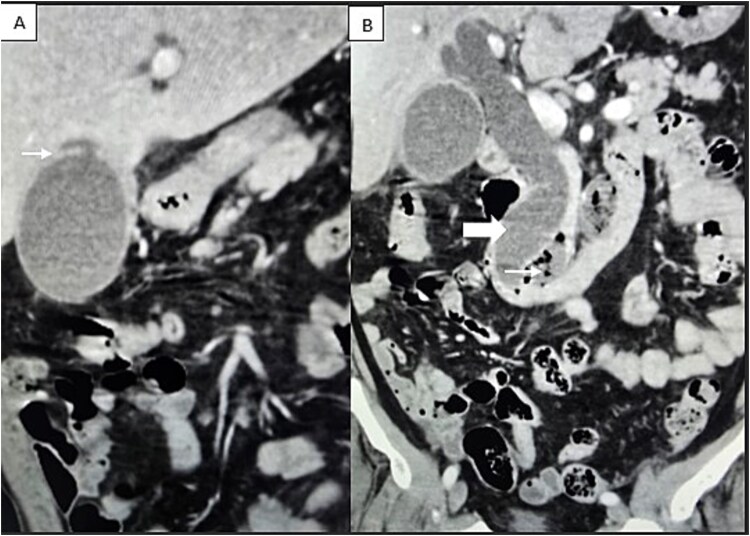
Contrast-enhanced CT scan demonstrating (A) localized perforation at the fundus of gallbladder (white arrow). (B) Extrahepatic biliary tract dilatation (big arrow); a periampullary duodenal diverticulum (small arrow).
